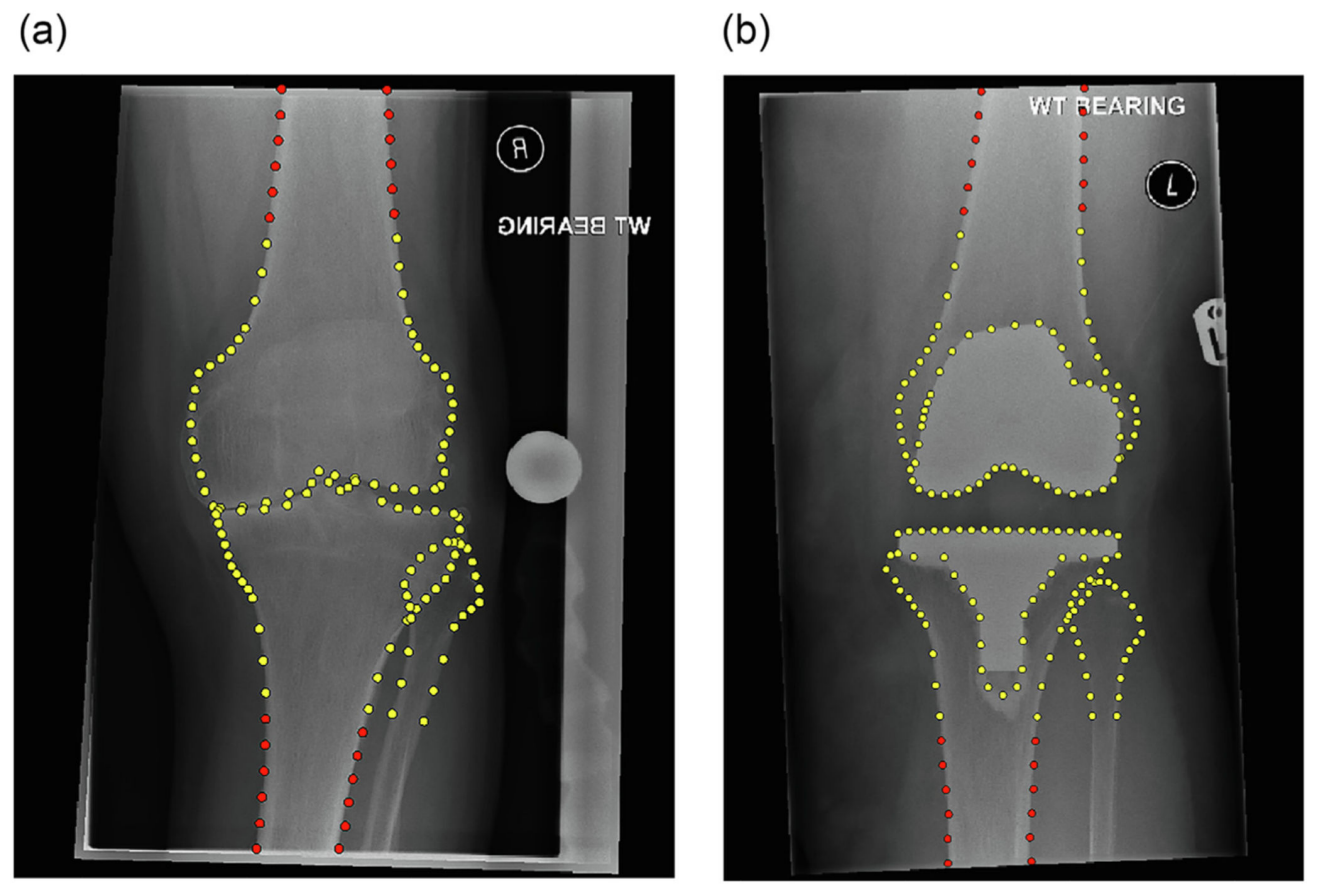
Annotation examples for the original/extended points sets (manually placed): (a) pre-operatively with 110/134 points, and (b) post-operatively with 157/181 points. The 24 extended points are highlighted in red. All radiographs have been cropped for better visualisation. Best viewed in colour. (For interpretation of the references to colour in this figure legend, the reader is referred to the web version of this article.)
